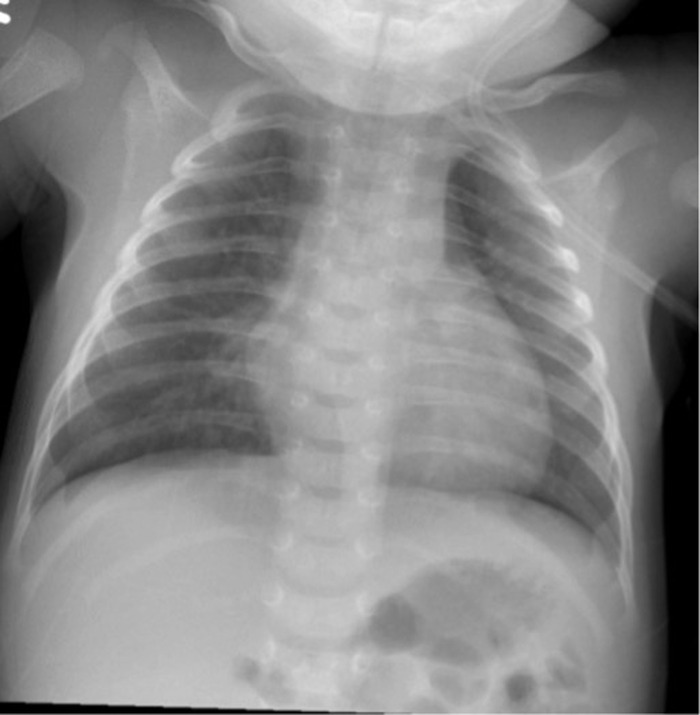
Chest radiograph showed bilateral perihilar hazy infiltrates that were greater on the right.
